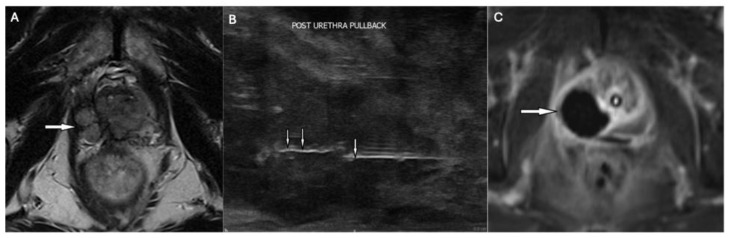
Pulsed electric field (PEF) ablation for recurrent right apical prostate cancer. (A) Transverse T2-weighted MRI shows a bilobed recurrence (arrow) one year post-focal laser ablation (Gleason 4 + 3). (B) Sagittal transrectal ultrasound during the salvage procedure. Microbubbles highlight the prior PEF needle track (double arrows), with the needle (arrow) retracted for a second activation (50 pulses). (C) Post-ablation contrast-enhanced axial T1-weighted MRI demonstrates an avascular ablation zone at the right apex (arrow), confirming accurate targeting.
